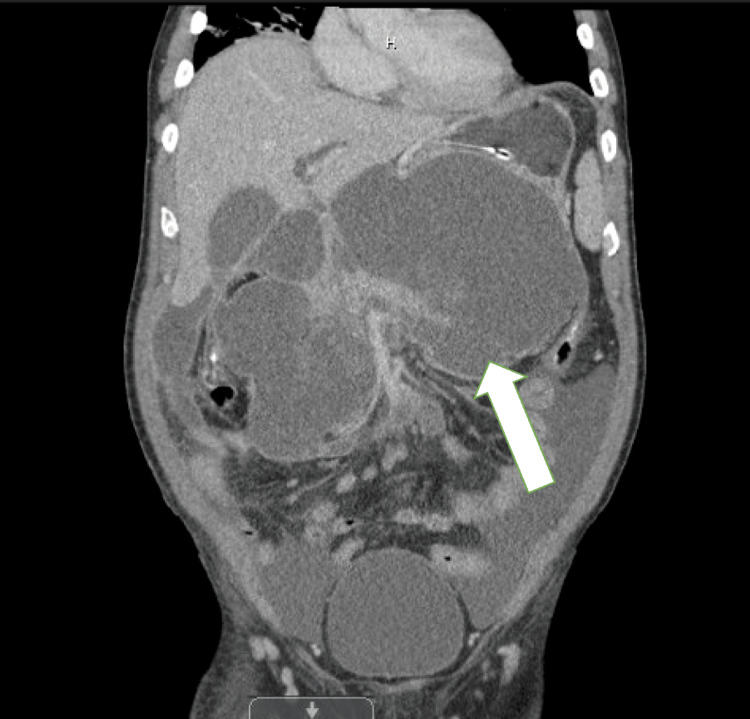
Coronal computed tomography image A large pancreatic pseudocyst (white arrow) is observed with clear delineation from the pancreas, causing a mass effect on adjacent abdominal structures.
